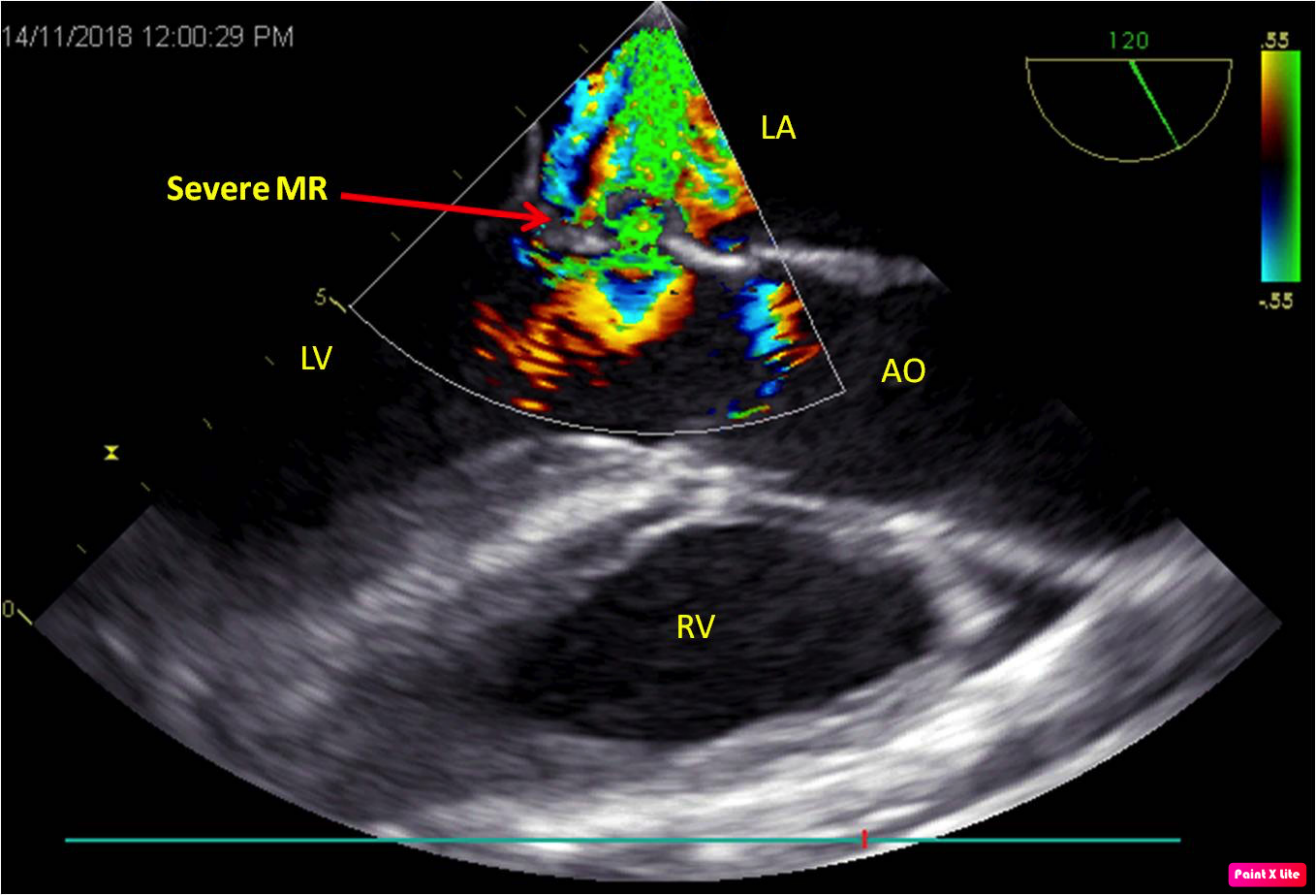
Transesophageal echocardiography showing perforation of anterior mitral leaflet with severe mitral regurgitation (MR). AO=aorta; LA=left atrium; LV=left ventricle; RV=right ventricle
